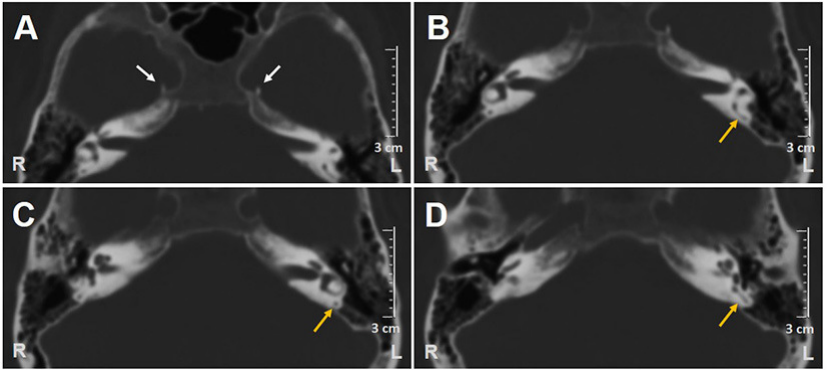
Axial scans of brain CT show (A) bilateral carotid siphon calcifications (white arrows) and (B–D) patent left PSC (yellow arrows). CT, computed tomography; L, left; PSC, posterior semicircular canal; R, right.
